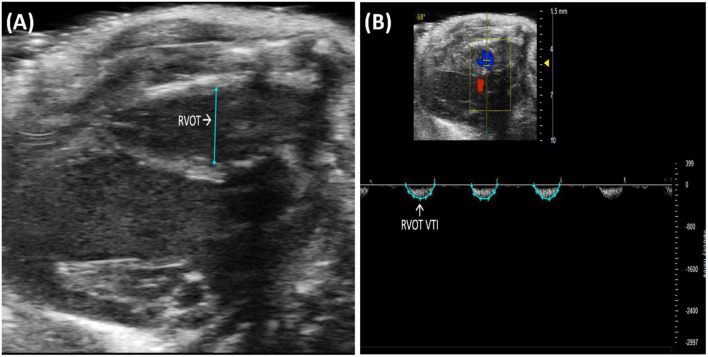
(A) Right ventricular outflow tract (RVOT) measures the size of the right ventricular outflow tract at the end of diastole and is acquired and analyzed in B-mode of the parasternal long axis (PLAX) view. (B) Right ventricular outflow tract velocity time integral (RVOT VTI) measures blood flow through the right ventricular outflow tract (RVOT) and is acquired by pulsed wave (PW) Doppler imaging of the RVOT in B-mode of the parasternal long axis (PLAX) view.
